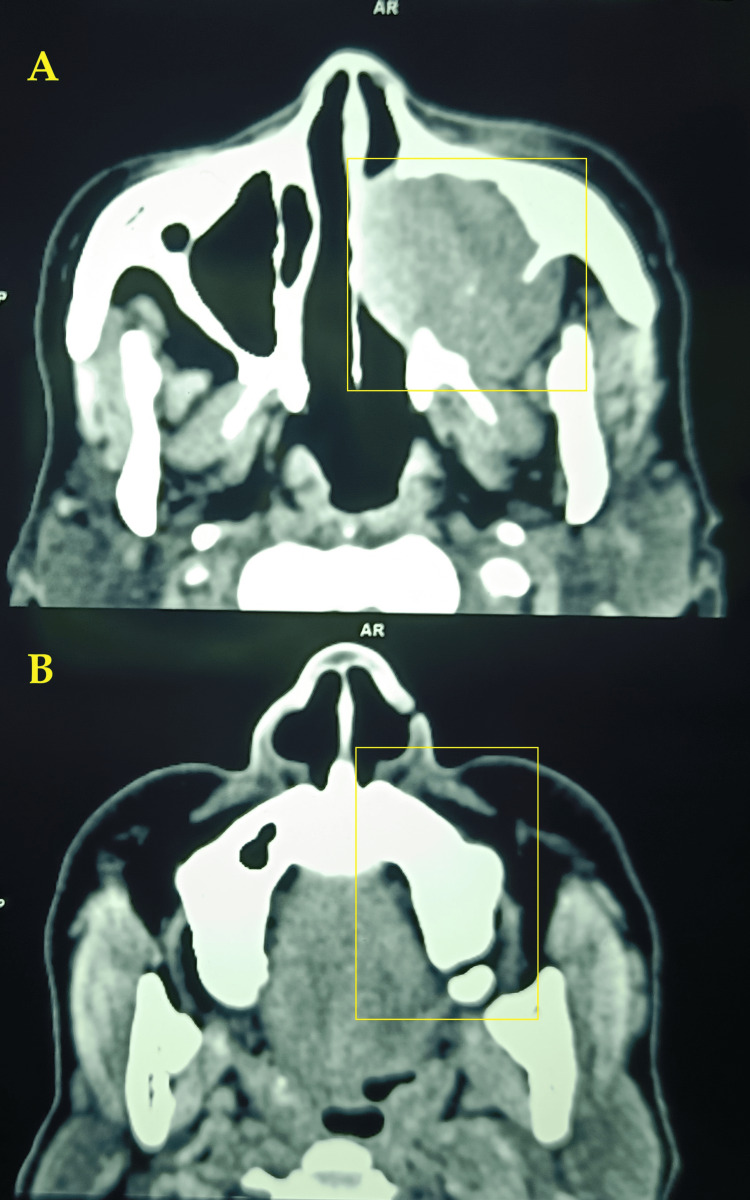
Contrast-enhanced computed tomography scan of (A,B) axial cuts showing the lesion in the left maxillary sinus extension into the left nasal cavity
